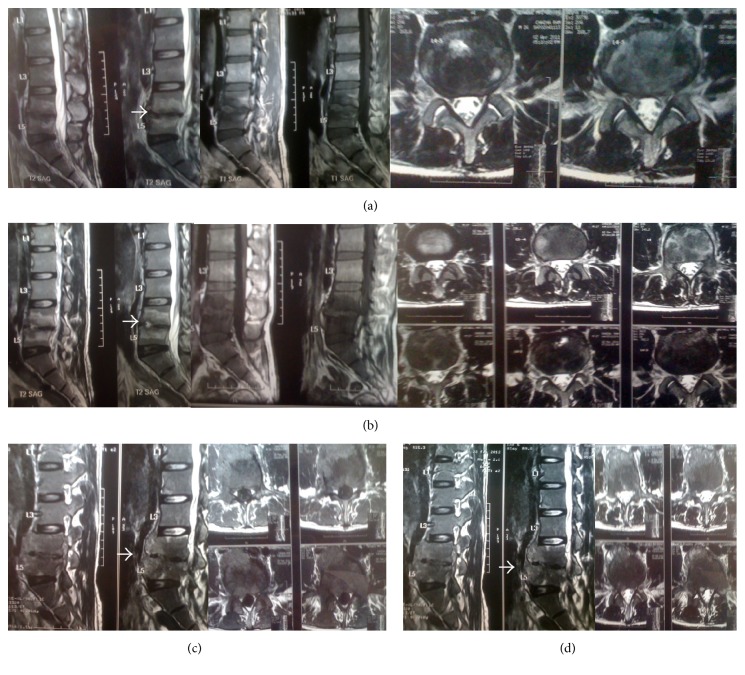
Sequential MRI images of the patient. (a) MRI before the initiation of anti-Koch's therapy. (b) MRI image after 4 months of anti-Koch's therapy. (c) MRI image after 9 months of anti-Koch's therapy. (d) MRI image after 12 months of anti-Koch's therapy.
